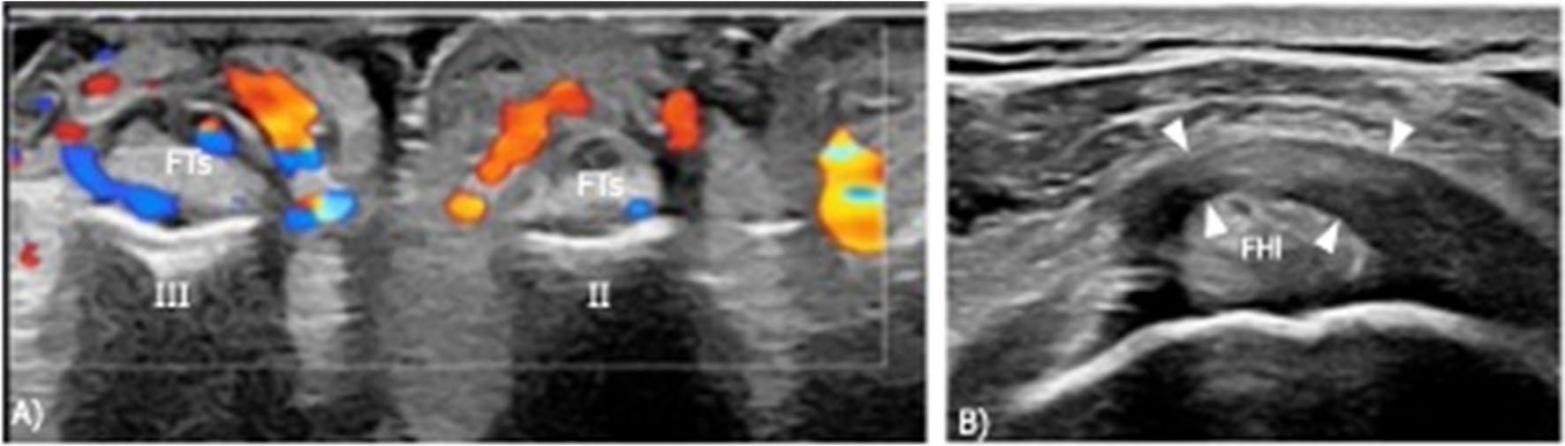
Case 2: Annular pulley thickening in inflammatory arthritis. Transverse US images of the ventral side of right forefoot obtained with a 18–4 MHz probe. A Signs of hypervascularization on Doppler imaging around the flexor tendons of the II and III toes. B Note the markedly thickened A1 pulley of the great toe (arrowheads). FTs, flexor tendons; FHl, flexor hallucis longus
